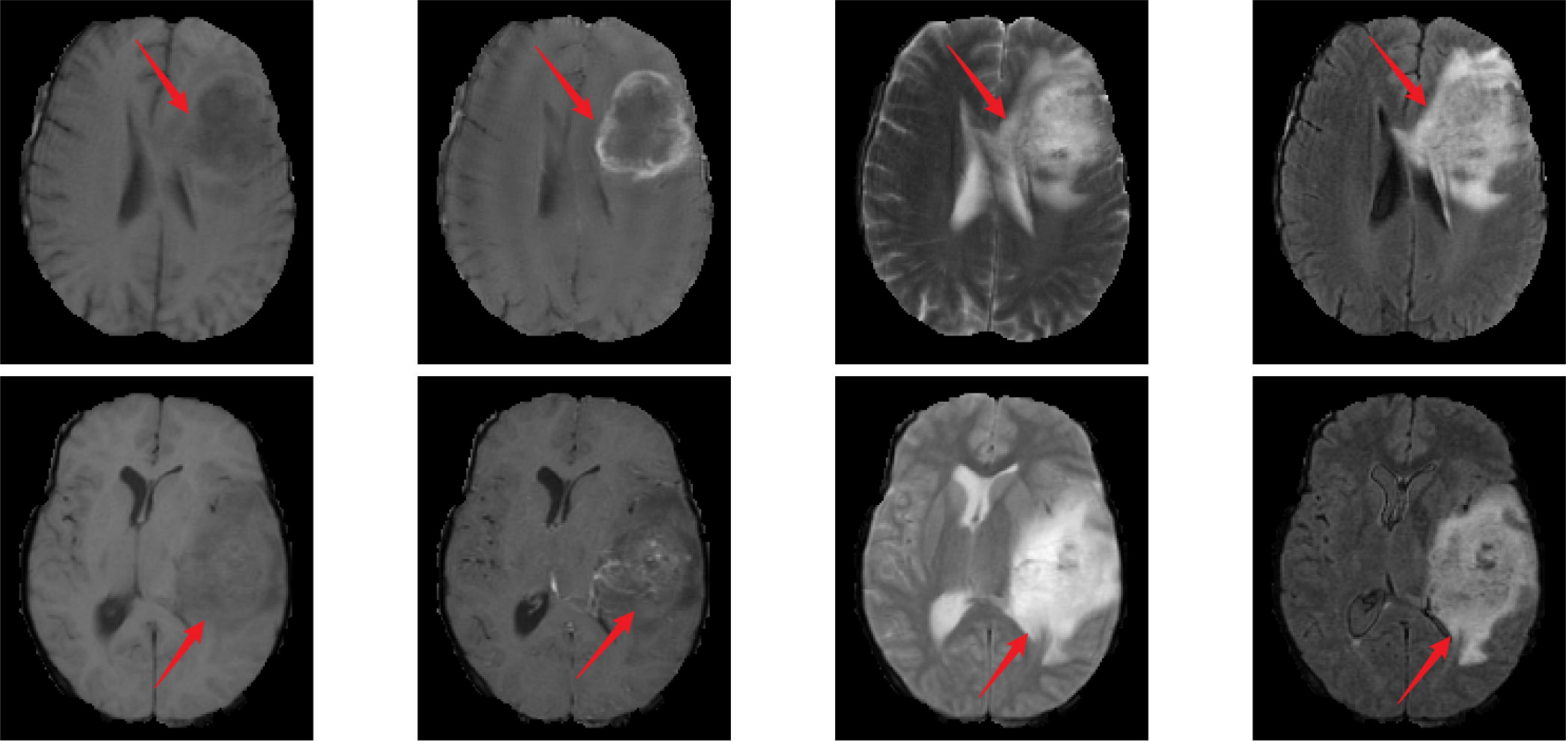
MR images of different modalities. From left to right, the images correspond to T1, T1-ce, T2, and FLAIR modalities. The first row represents cases with IDH wild-type, ATRX wild-type, and 1p/19q non-codeletion status. The second row represents cases with IDH mutant, ATRX mutant, and 1p/19q non-codeletion status.
